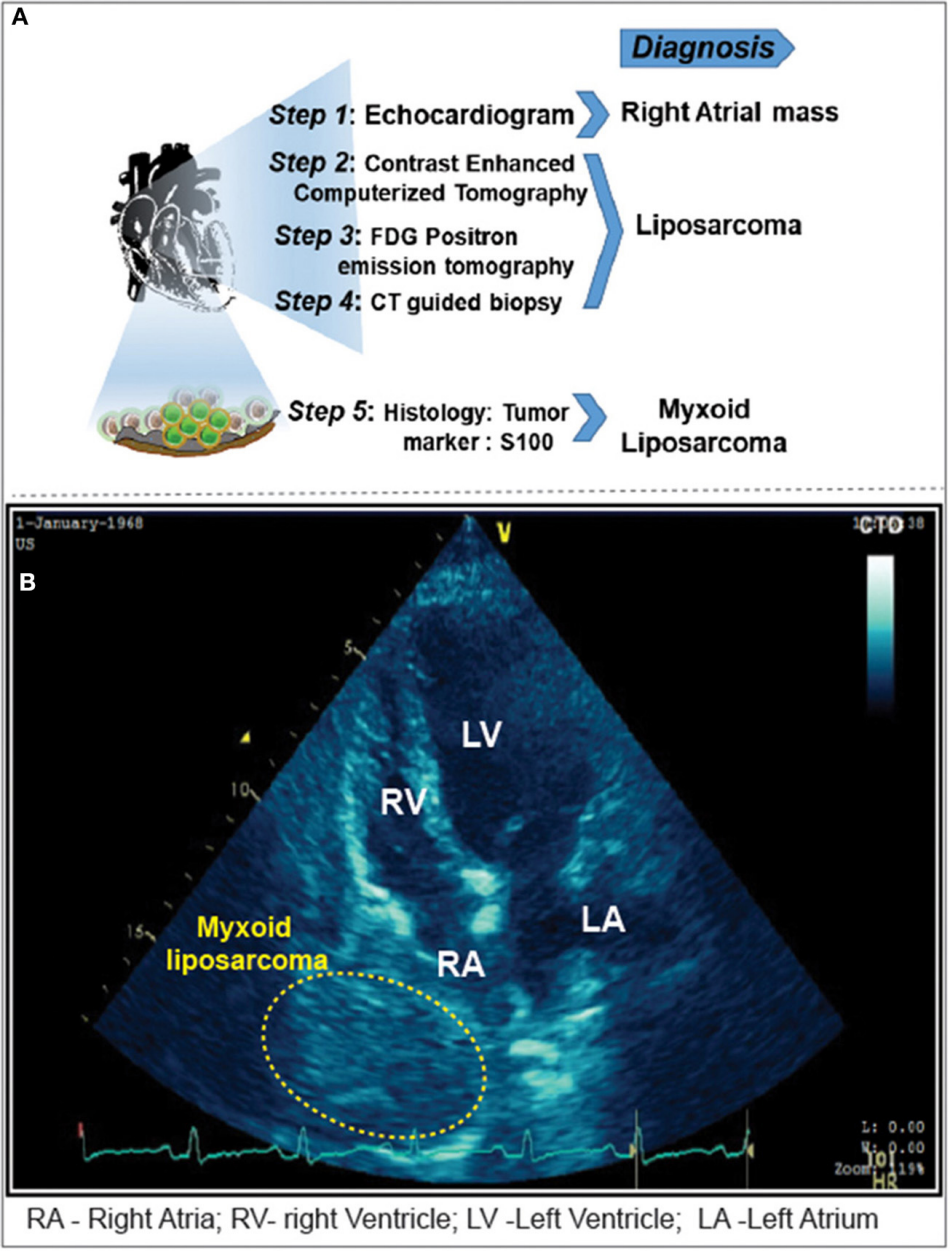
(A) Imaging modalities and approaches carried out for the diagnosis of cardiac tamponade. (B) Pericardiocentesis followed by 2D-Echocardiogram revealed a 5 x 4 cm sized mass adjacent to the right atrium. Yellow highlighted area locates the myxoid liposarcoma in the right atrium.
